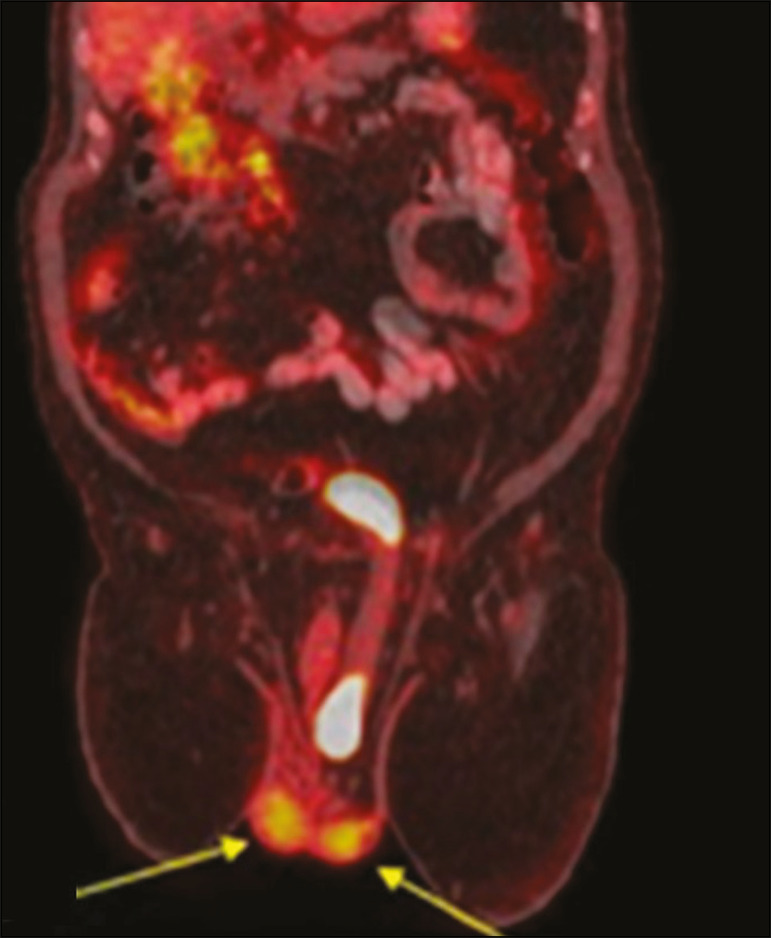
^18^F-FDG-PET/CT fusion image showing the normal testicles (arrows) and a left inguinal hernia containing the bladder, in which there was physiological accumulation of the radiopharmaceutical.
